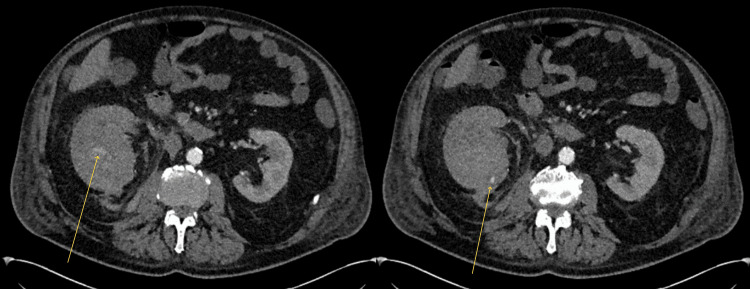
CT abdomen (axial view) in the arterial phase The CT images show dense contrast within the haematoma around the lower pole of the right kidney measuring 9.5 x 7 x 10 cm (arrows), suggestive of active bleeding. The bleeding is possibly from one or more subcapsular branches of one of the right renal arteries. CT, computed tomography
